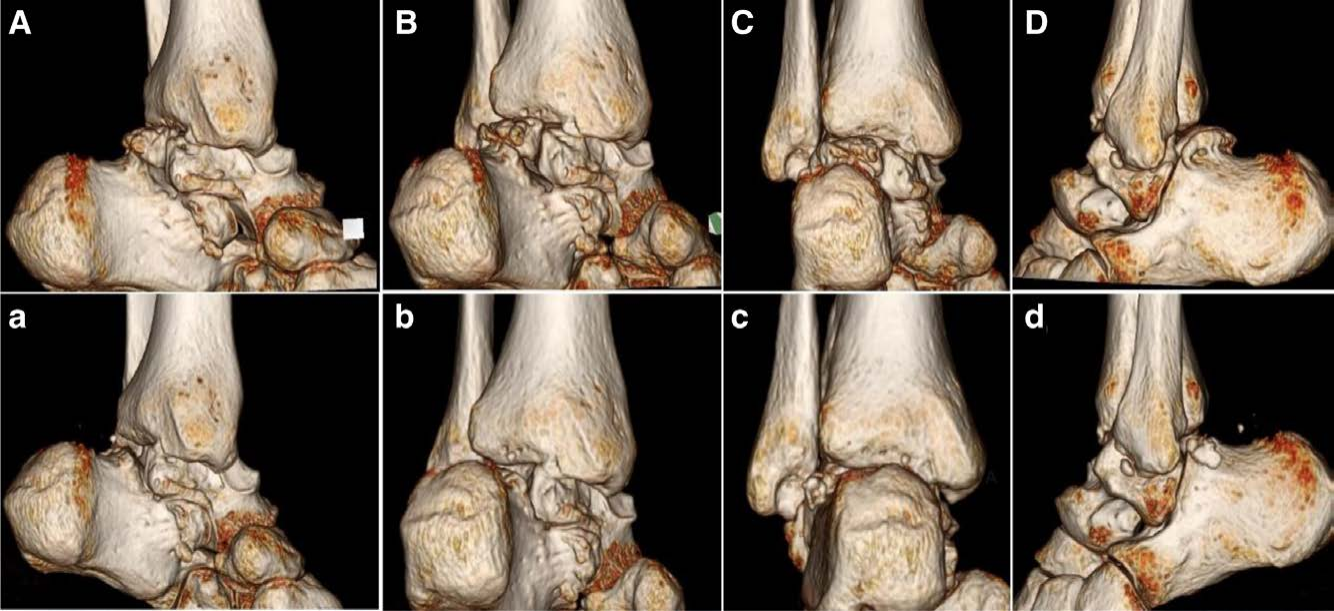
(A–D) Preoperative CT 3D scan images clearly show the bipartite talus and its size. (a–d) Postoperative CT 3D scan images show that the bipartite talus has been basically removed. CT = computed tomography.
